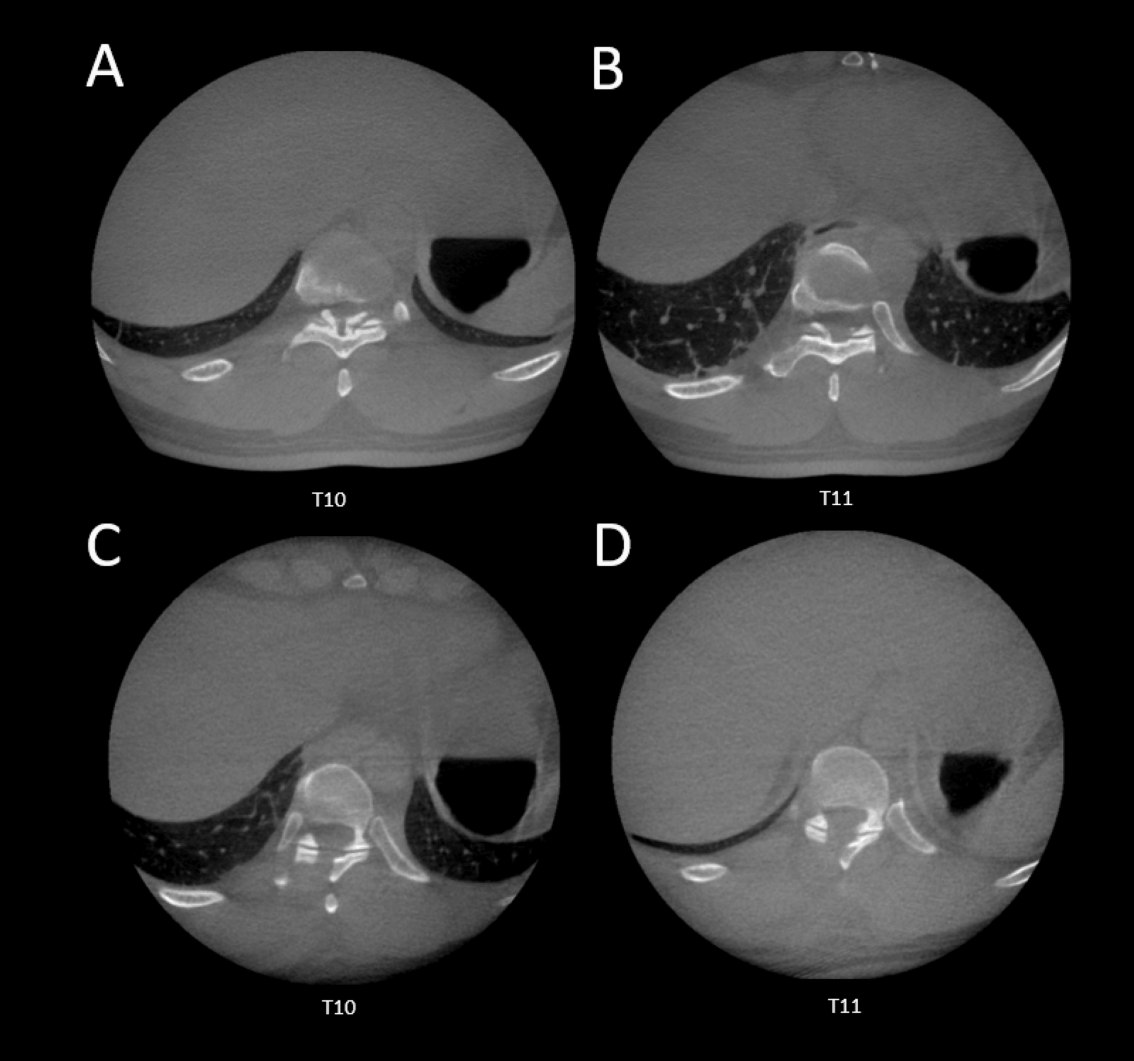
(A-B) Intraoperative CT scans demonstrating the extent of the OYL. (C-D) Postoperative CT scans showing complete removal of the OYL. OYL: ossification of the yellow ligament
